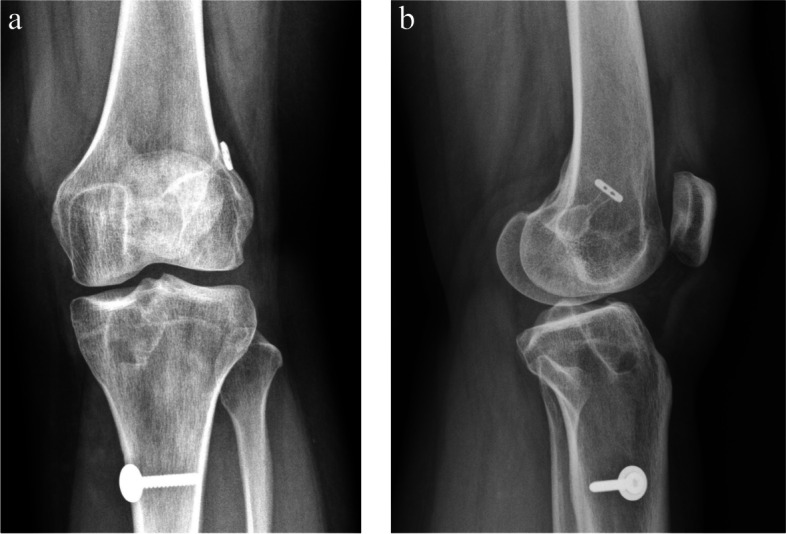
a Anteroposterior view of radiograph of CSIS hybrid tibial fixation at least 1 year after operation. b Lateral view of radiograph of CSIS hybrid tibial fixation at least 1 year after operation
